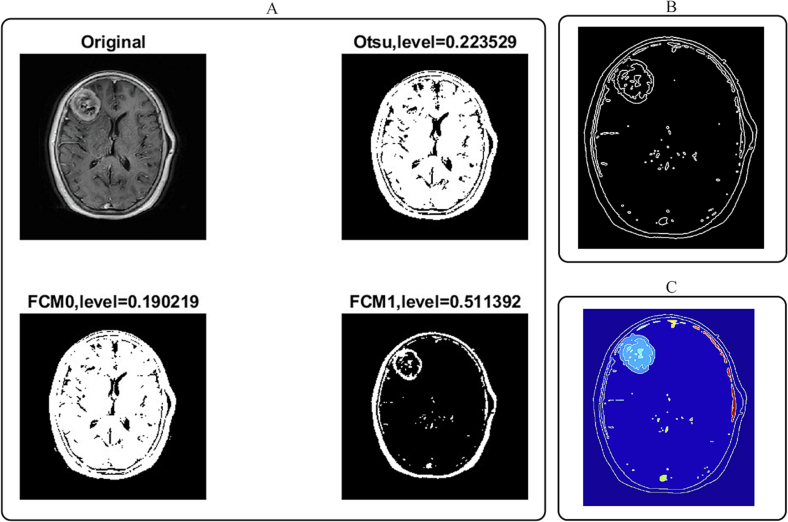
Segmentation process of meningioma in axial CE T1-weighted MRI images of patient 3 (A: including the original image and thresholding measurements using Otsu's and FCM clustering; B: gradient magnitude image; C: color map image).
